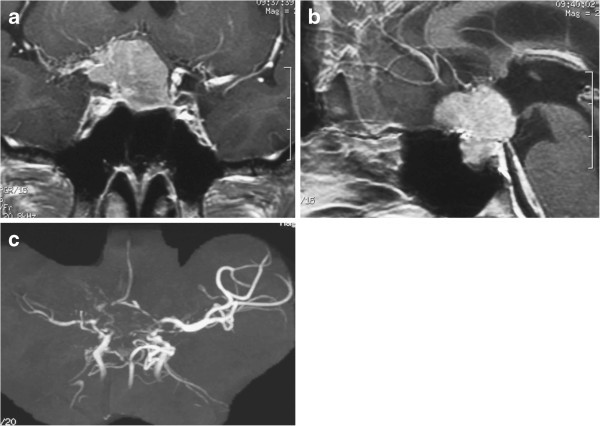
Case 1. Coronal (a) and sagittal (b) head MR images with contrast medium showing a well-demarcated skull base tumor, and abnormal mesh-like enhancement around the right ICA terminal and at the distal portion of the ACA. MR angiogram showing severe stenoses of the bilateral ICA terminals and moyamoya vessels (c).
